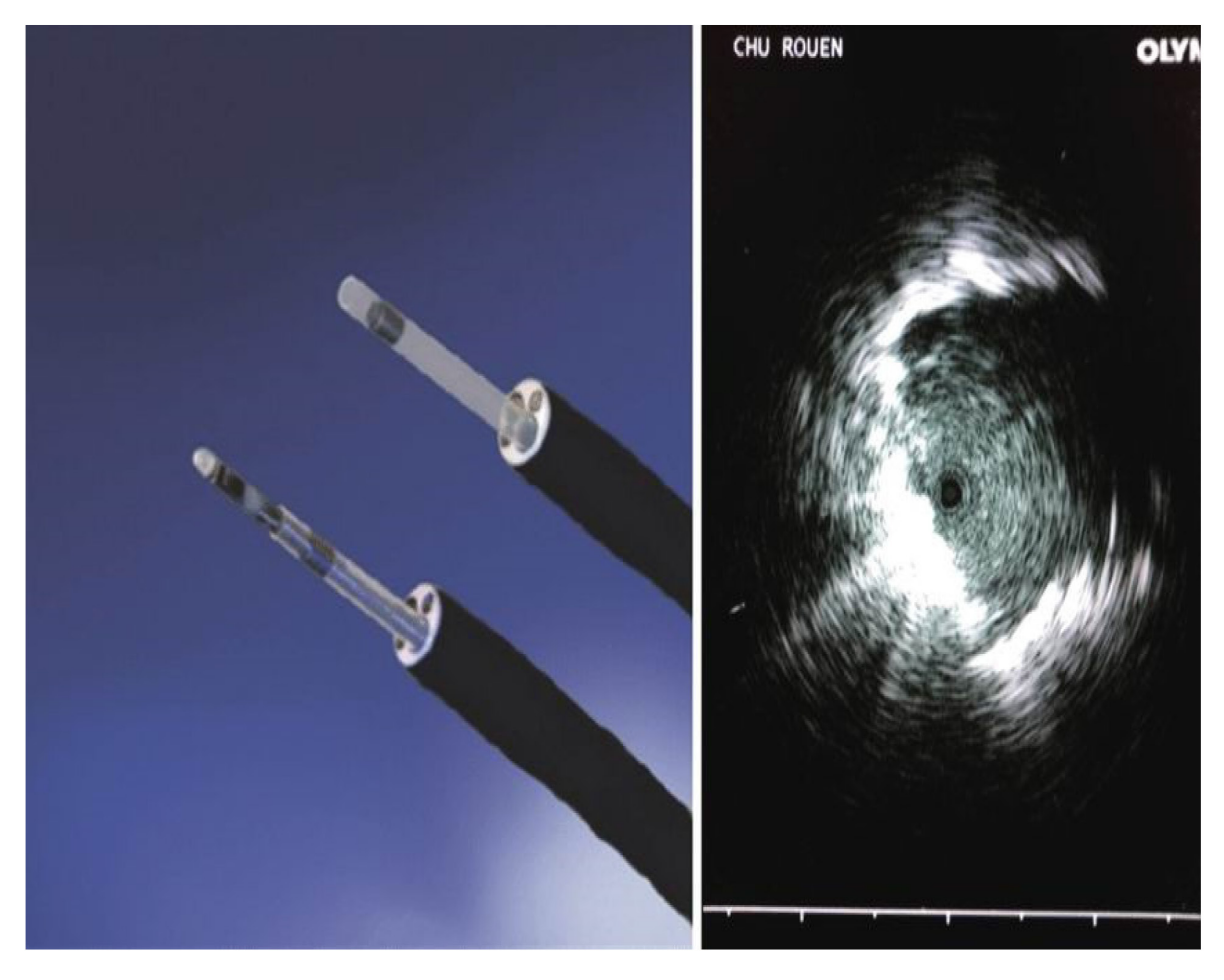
Radial ultrasound probe UM-S20-17S (1,4mm) and UM-S20-20R-3 (1,7mm w/o guide sheath).
